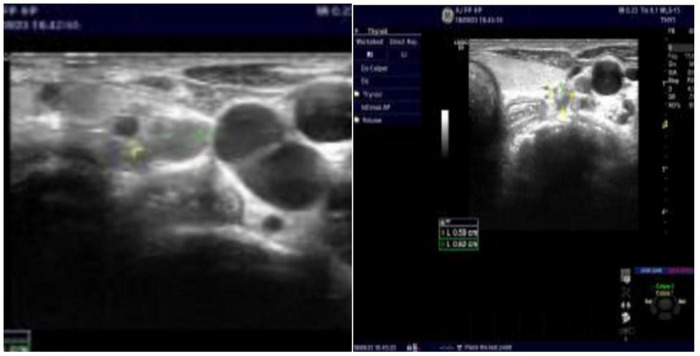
B-ultrasound image before parathyroid radiofrequency ablation.
